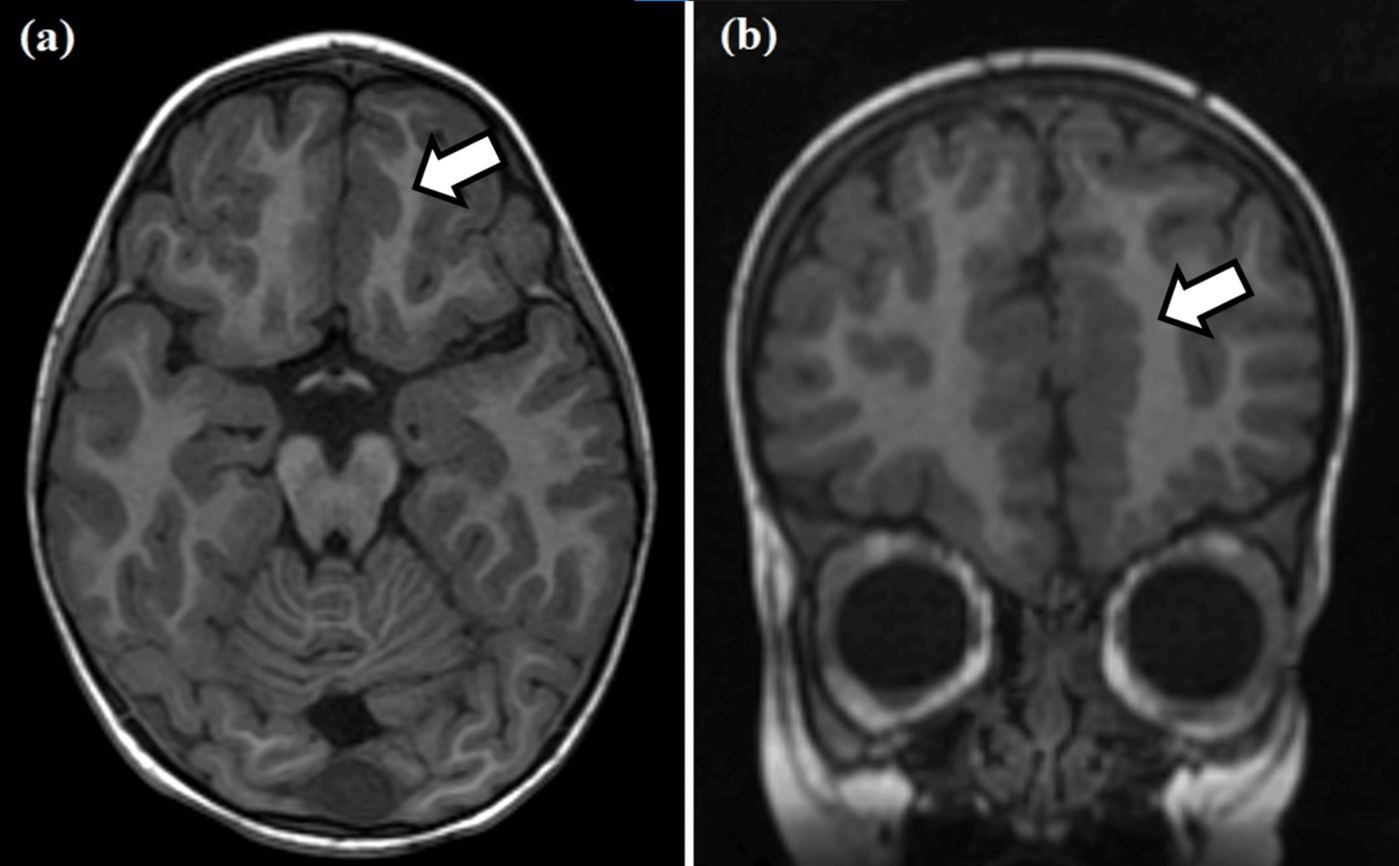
Axial (a) and coronal (b) T1-weighted MRIs. Cortical thickening and micro-lobulation at the cortico-subcortical interface (white arrows) are noted in the mesial surface of the left frontal pole, specifically at the transition from the superior frontal gyrus to the gyrus rectus.
